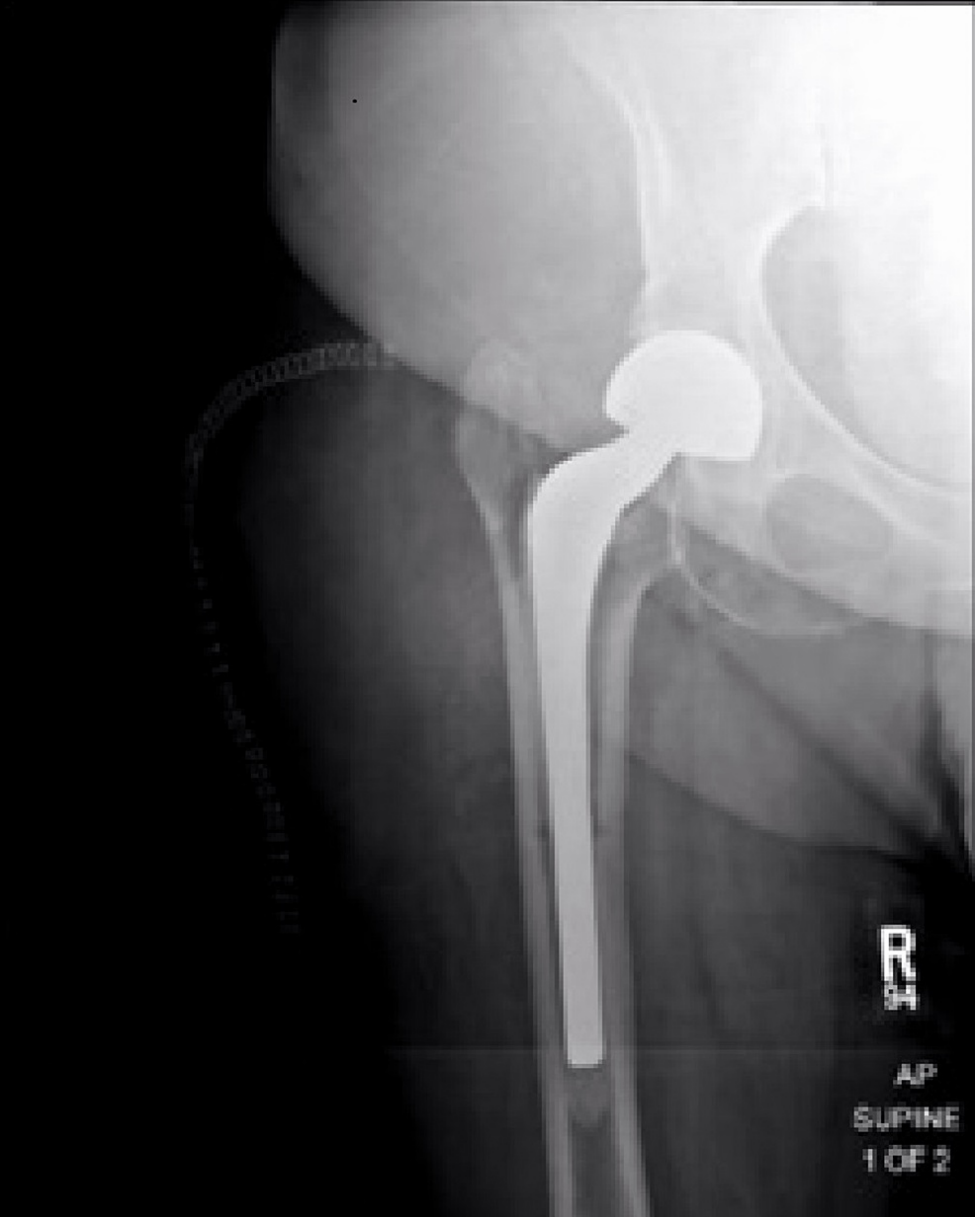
AP radiograph of the right hip after presenting with intractable hip pain two weeks status post placement of the PROSTALAC spacer There is concern for a periprosthetic femur fracture with subsidence of the femoral stem compared to the previous radiographs.
